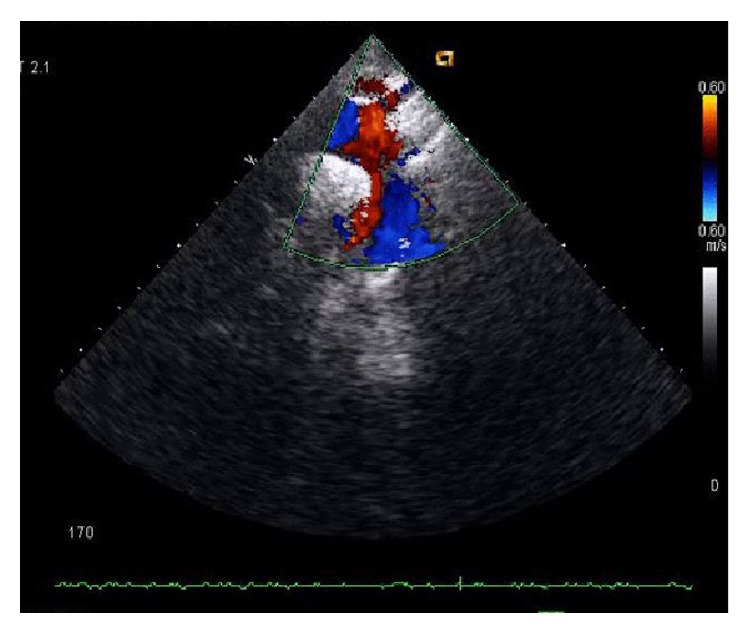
ECHO-Doppler study showing stenosis of the right subclavian artery.
